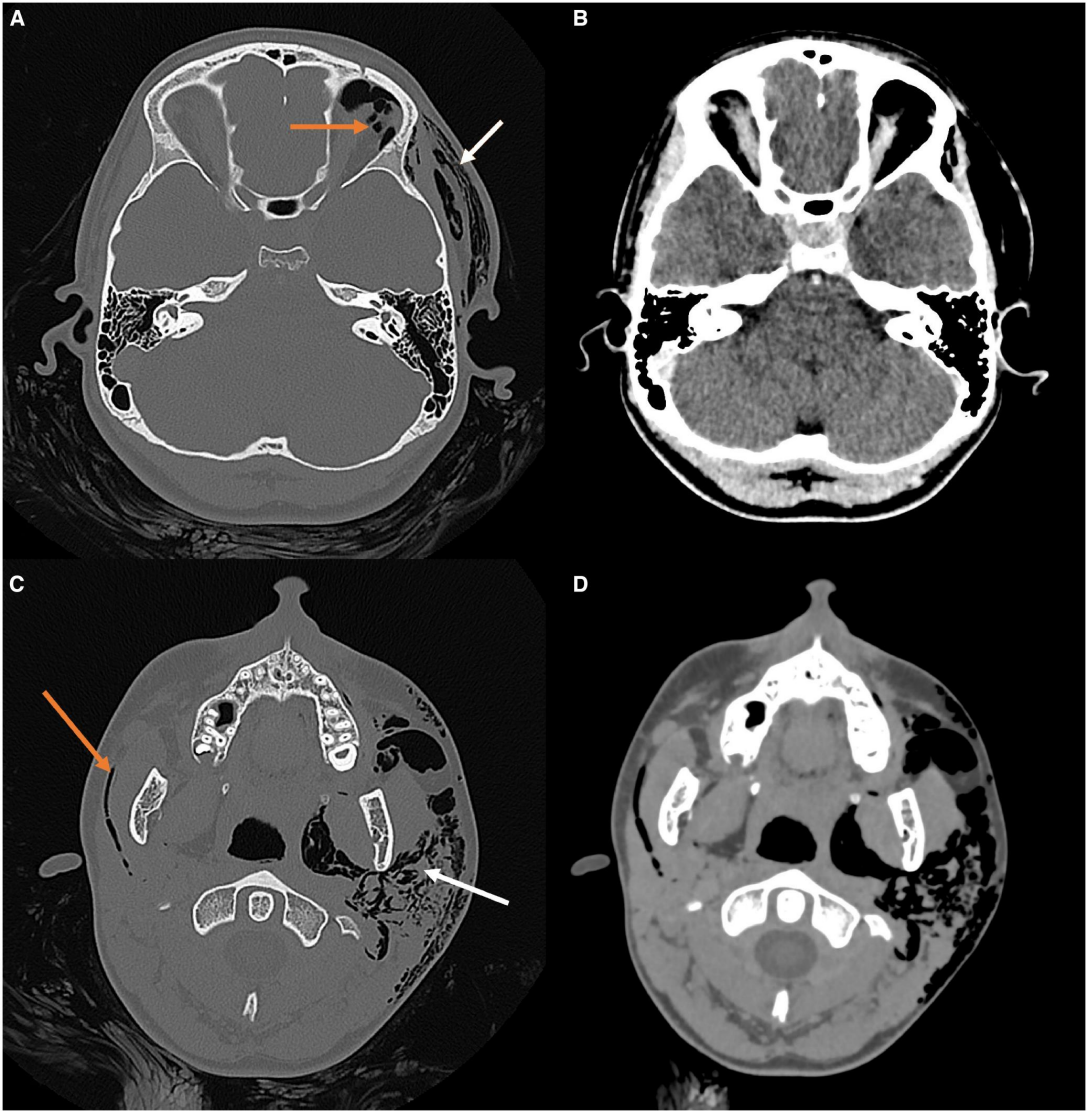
Non-contrast CT orbits. (A) Subcutaneous gas seen in the soft tissues of the left scalp (white arrow) and left periorbital and extraconal spaces (orange arrow). (B) No other brain or soft tissue abnormality evident on brain windows of the same slice. (C) Extensive gas disseminated throughout the left parotid gland (white arrow), dissecting into the adjacent subcutaneous tissues. Notably, there is gas delineating the right Stensen’s duct (orange arrow). (D) On soft tissue windows of the same slice, the right parotid gland was normal.
